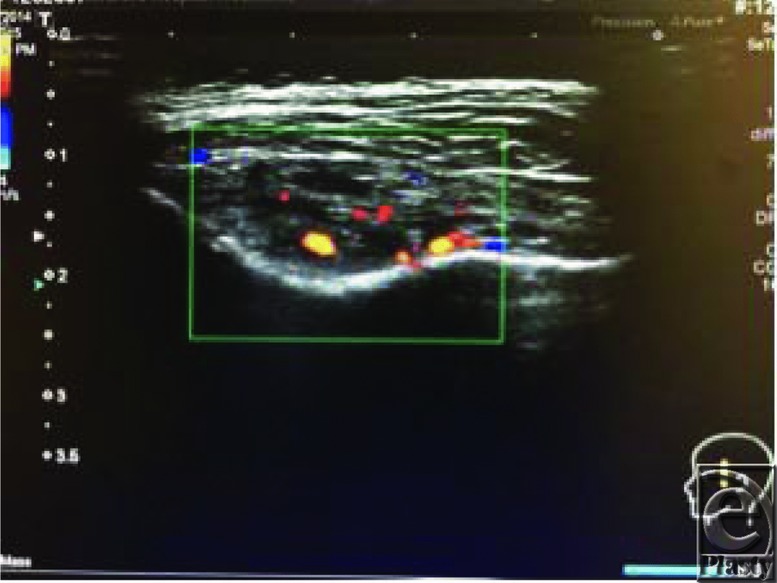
Ultrasound showing heterogeneous mass with no increased vascularity on duplex scanning.
